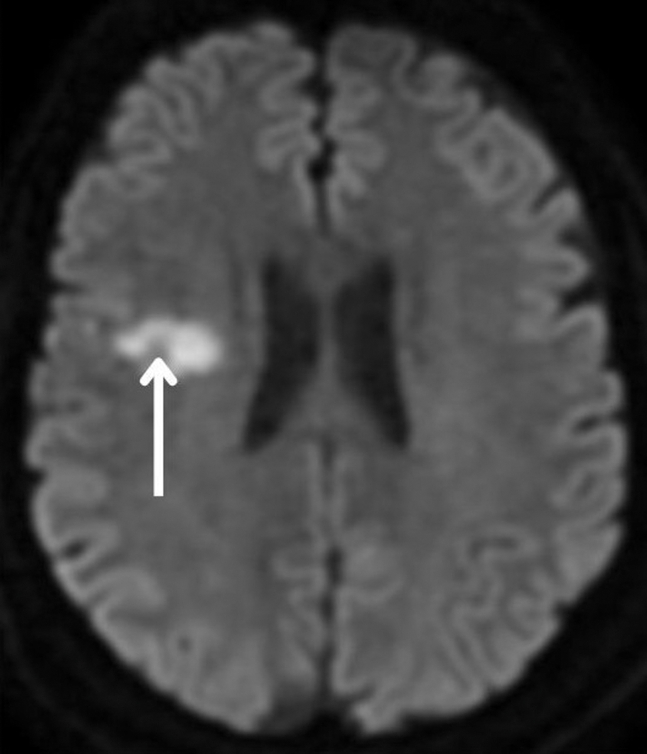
Magnetic resonance imaging revealing high signal intensity in the right frontal lobe (arrow), consistent with acute ischemia.
